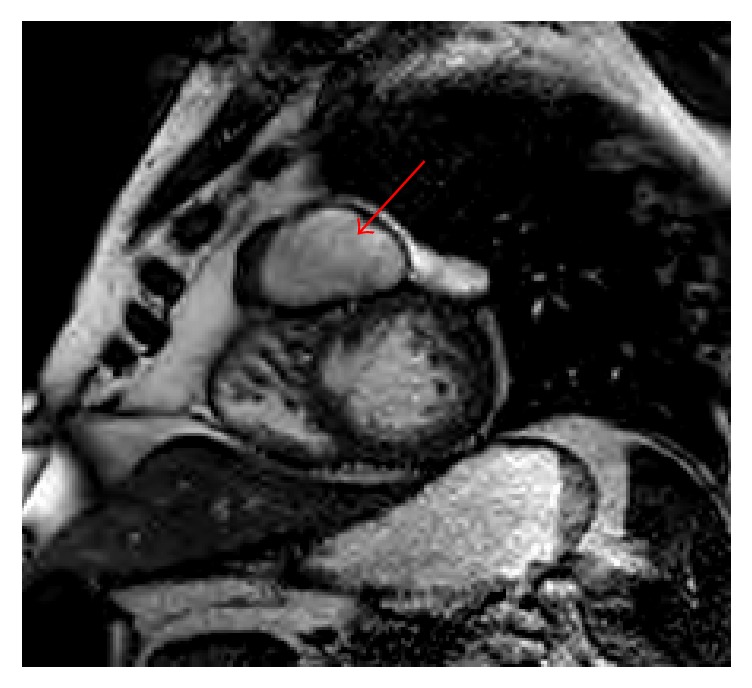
Cardiac MRI.  5.4 × 4.1 × 4.9 cm pseudoaneurysm arising from the mid-apical free wall of the right ventricle, with a 9 mm neck (red arrow). There is an adjacent muscular ventricular septal defect, which is likely related.
